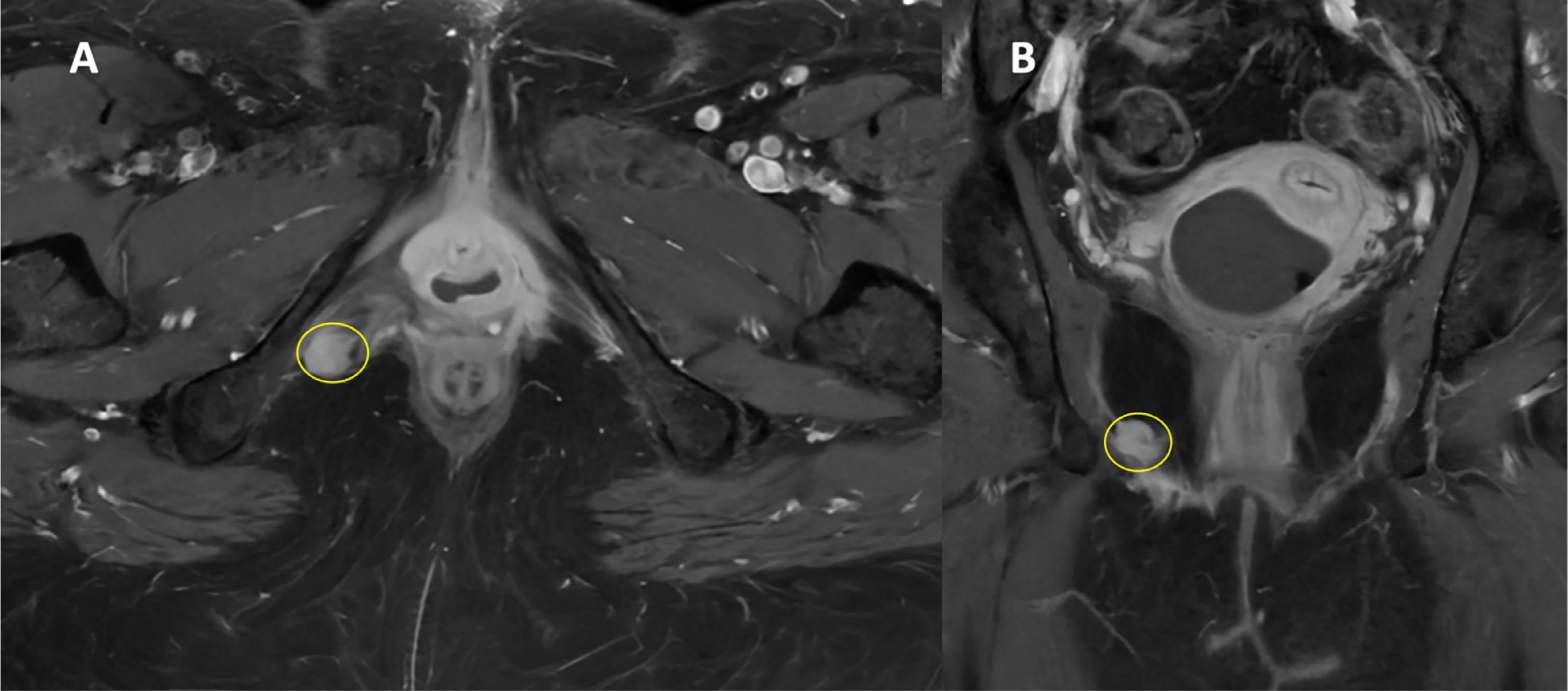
MRI scan T1w with gadolinium contrast, performed to assess for local recurrence, showed a 1 cm contrast enhancing hyperintense lesion at the pudendal nerve route without invading the side wall, muscles and bones. May 2023, pre-SABR. (A, axial view; B, coronal view).
